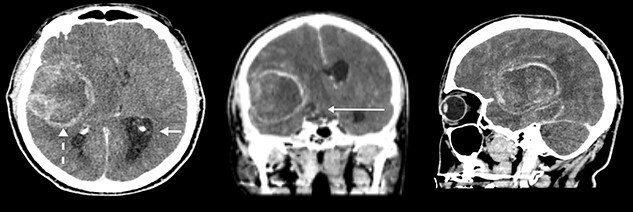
(A–C) Contrast CT at presentation (axial, coronal and sagittal) demonstrating a large right temporal lesion and peri-lesional oedema (dashed arrow), contralateral hydrocephalus (short straight arrow), causing significant mass effect, including uncal and subfalcine herniation (long straight arrow).
